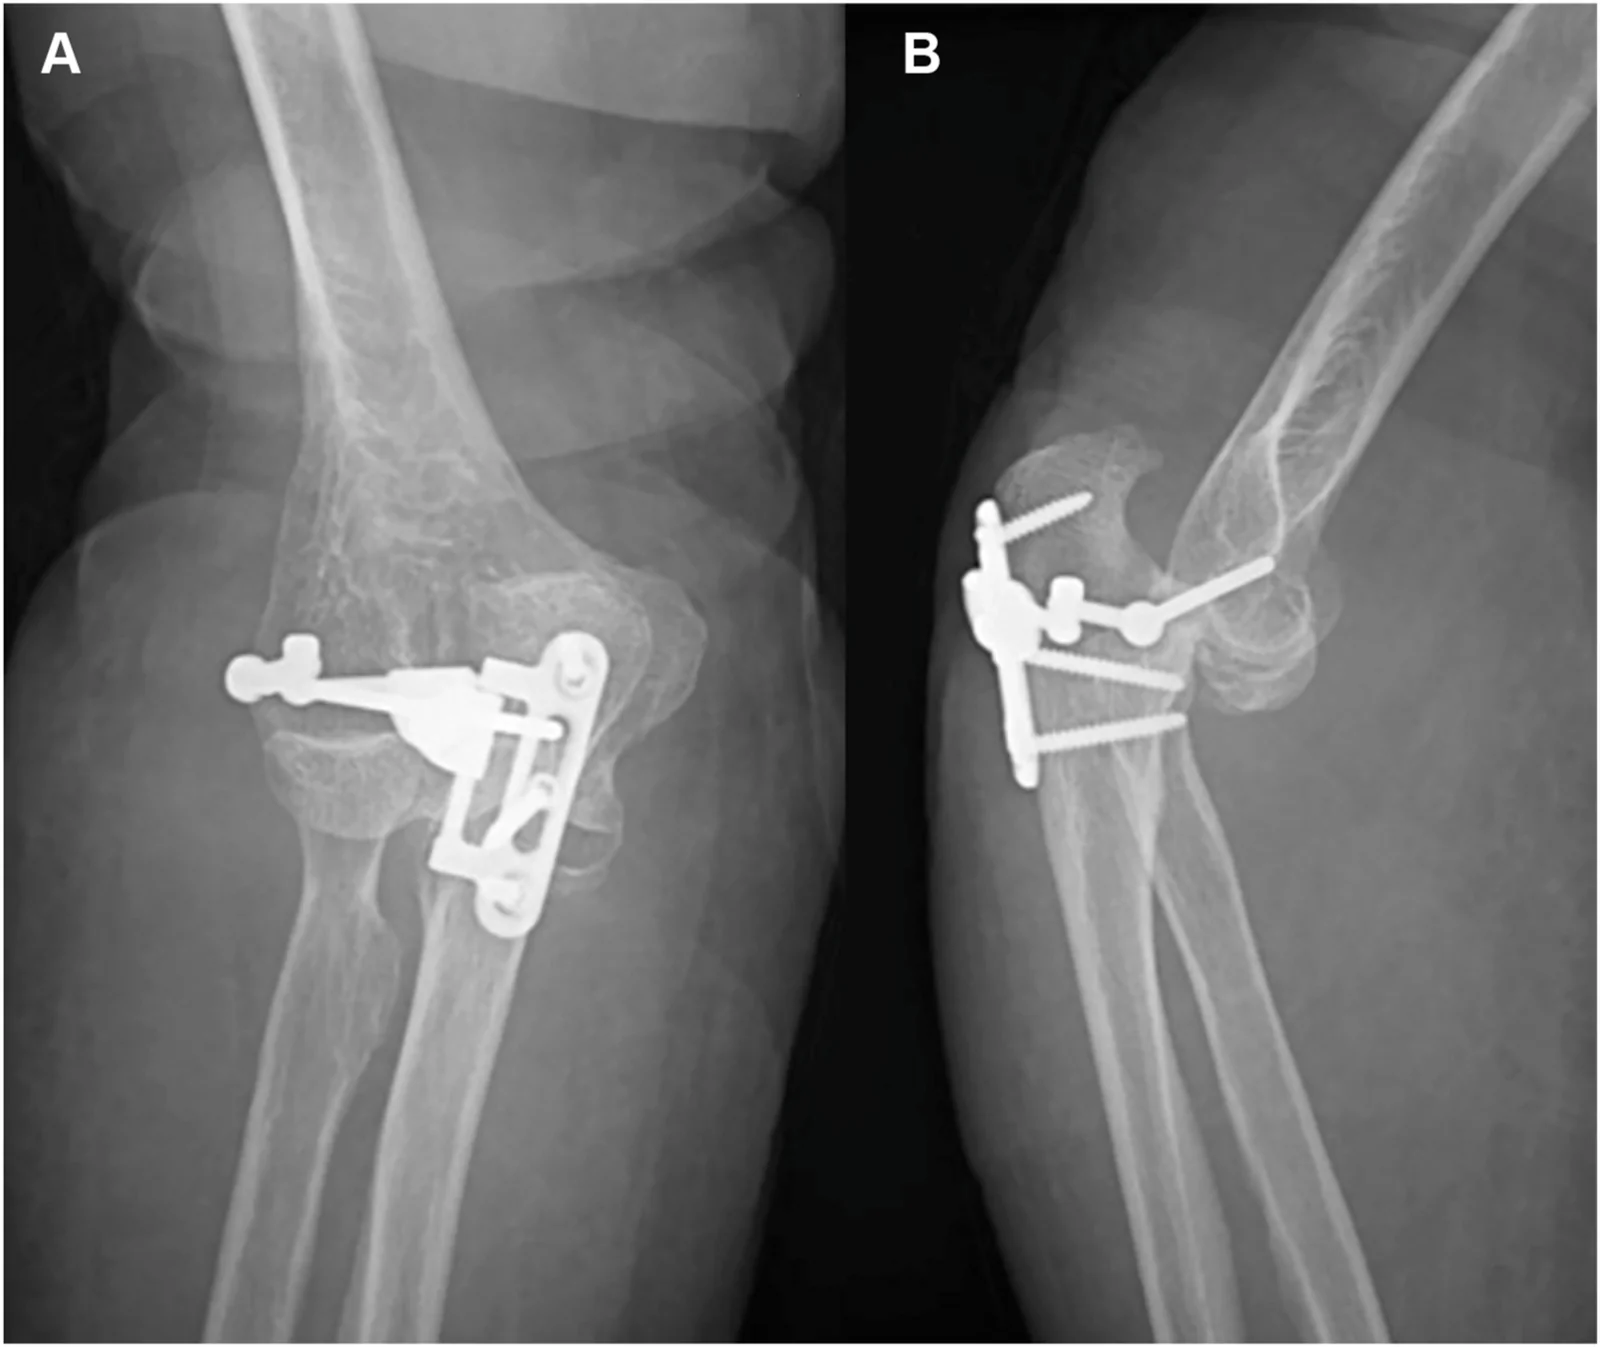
Antero-posterior (A) and lateral (B) radiographic imaging of recurrent elbow dislocation in the post-operative period of open reduction and internal fixation with an internal joint stabilizer.
